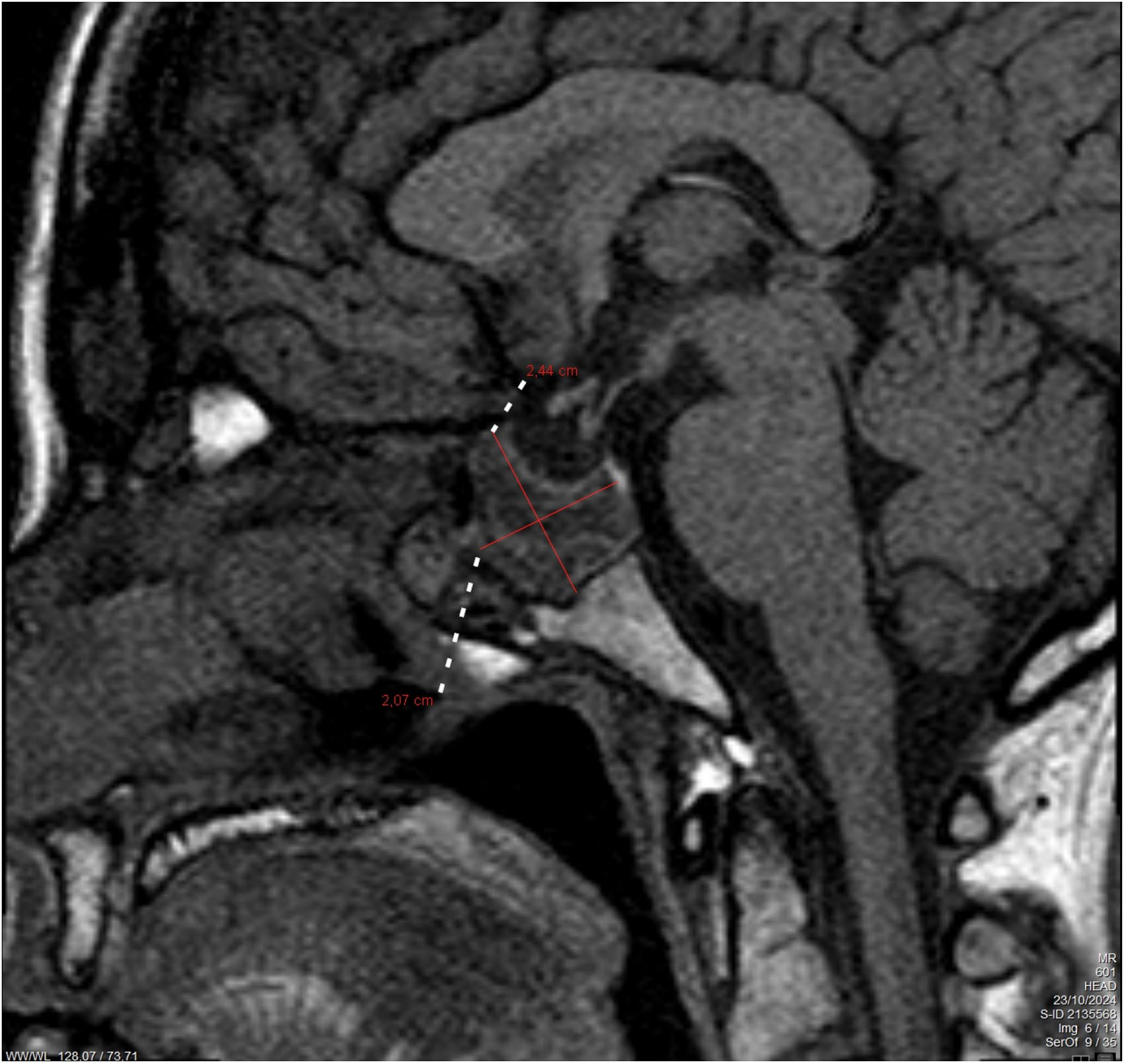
Follow-up brain magnetic resonance imaging (MRI) after 4 months of delivery. The figure shows a slight decrease in the hemorrhagic macroprolactinoma with dimensions of 24.4 × 20.7 mm (marked with red lines).
